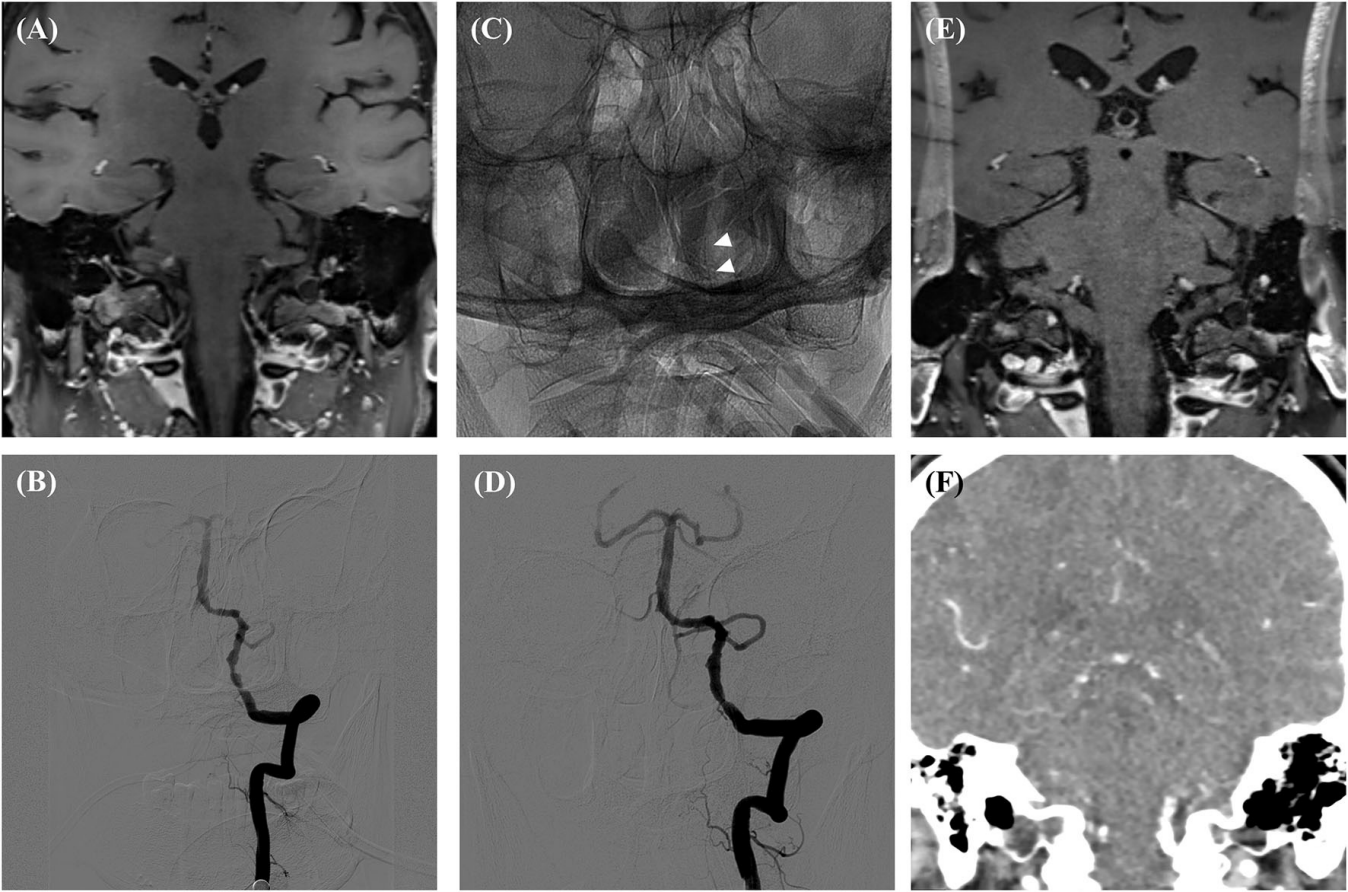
Preprocedural HR-MR (A), Preprocedural DSA (B), DSA immediately after the procedure (C,D), follow-up HR-MR (E) and CTA (F). There was a signal drop at the level of the vessel in the follow-up HR-MR images. White arrows showed the Apollo stent.
